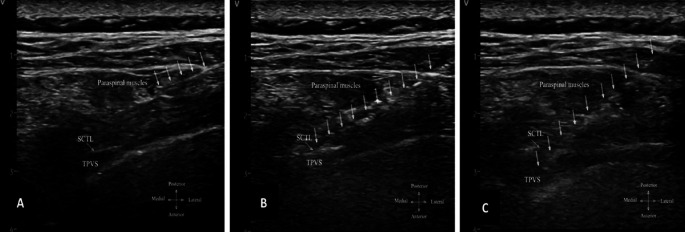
Ultrasound image of thoracic paravertebral block and needle tip position during pressure measurement. (A) Paraspinal muscle. (B) Immediately posterior to SCTL. (C) In TPVS. Abbreviation: SCTL, superior costotransverse ligament; TPVS, thoracic paravertebral space; dotted arrow represents the needle path.
